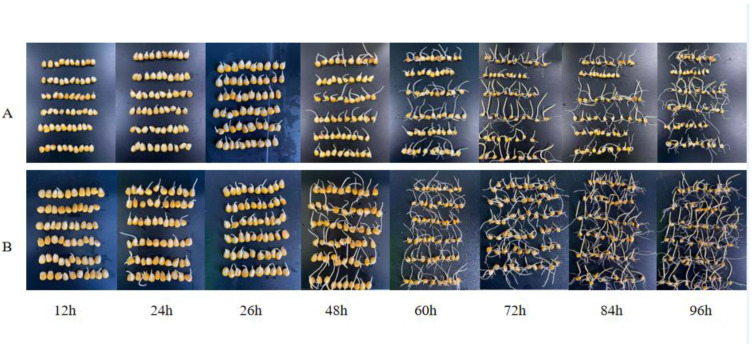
Different stages of germinated maize. (A) Control group. (B) Ultrasound group.
